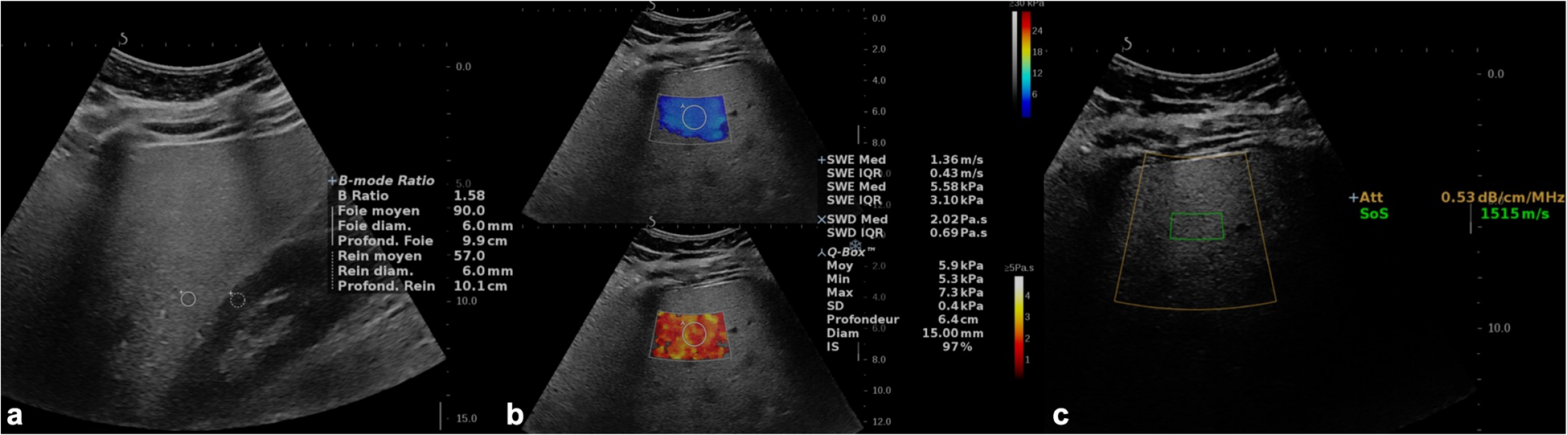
40-year-old man with hepatic steatosis. Multiparametric ultrasound assessment includes (a) hepatorenal index, (b) shear wave elastography imaging, and (c) attenuation imaging coefficient and sound speed estimation
